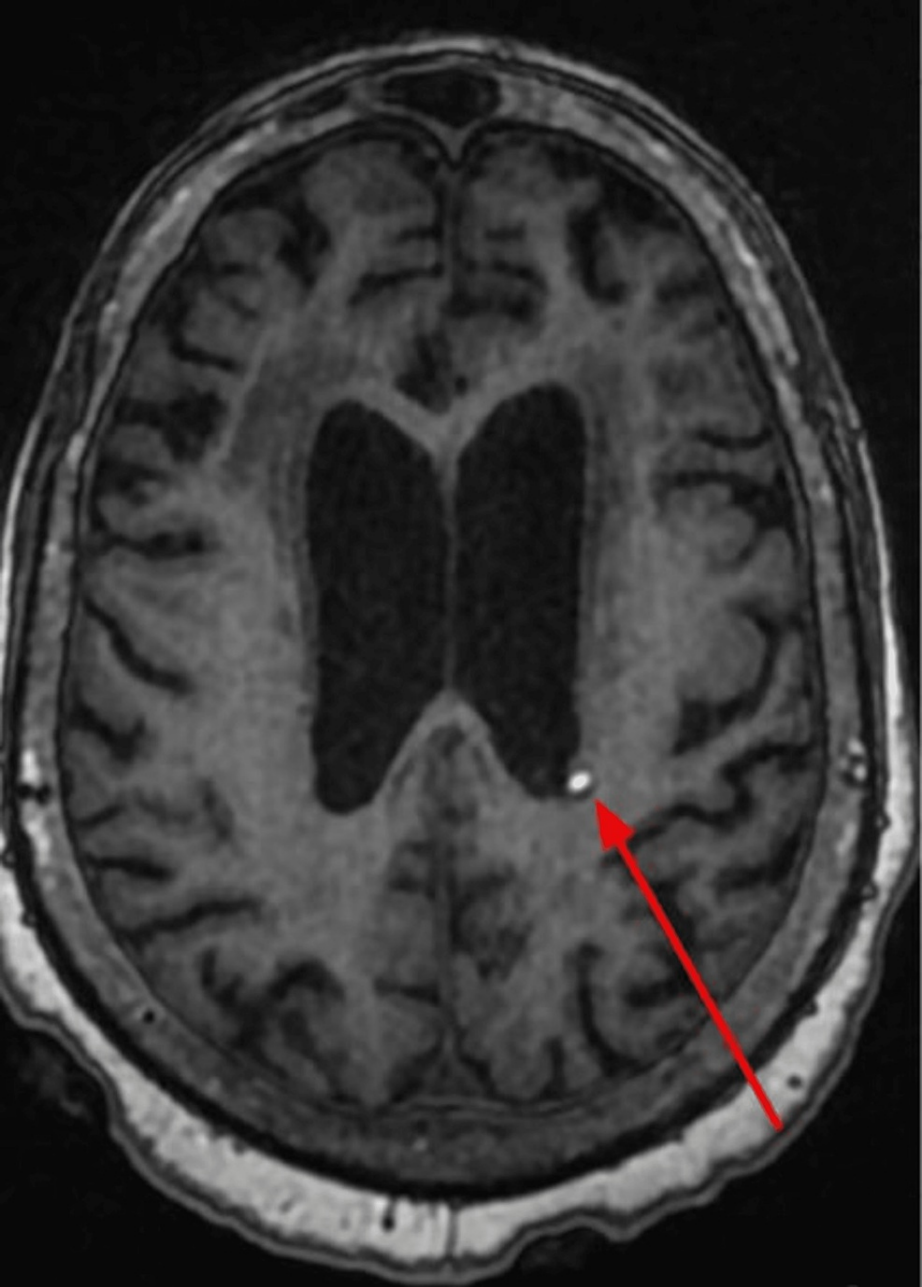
MRI brain T1-weighted image showing increased T1 signal in the white matter adjacent to the left lateral ventricle (0.5 cm). These findings are most compatible with a focus of subacute hemorrhage.
